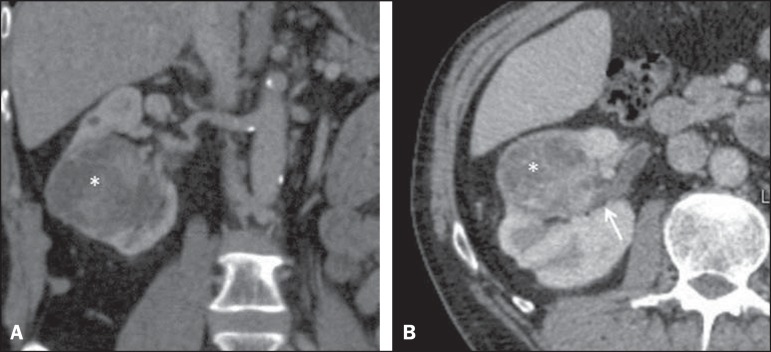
Coronal (A) and axial (B) CT images showing a
